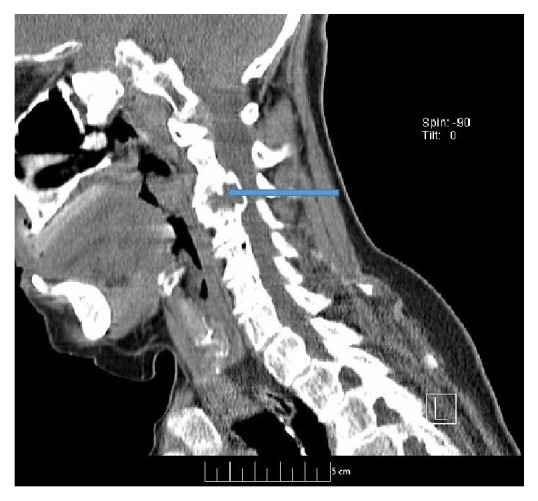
Sagittal CT of cervical spine image showing expansile lesion at C3 with preserved surrounding cortex.
